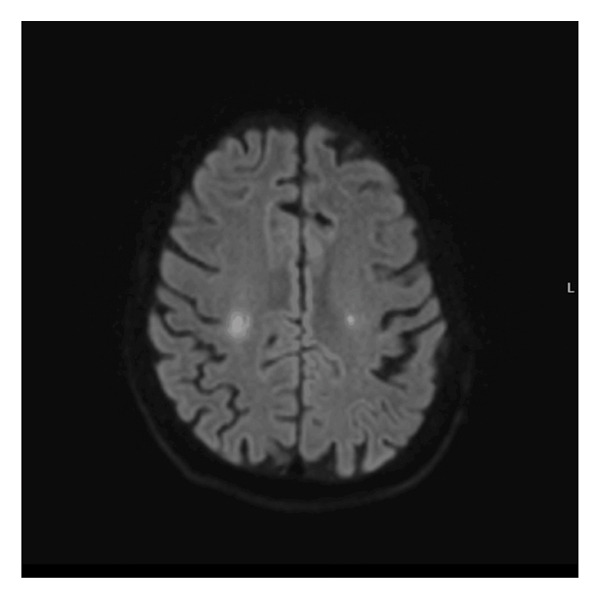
MRI of the brain with ischemic lesions consistent with septic emboli and microabscesses bilaterally in the semioval center.
